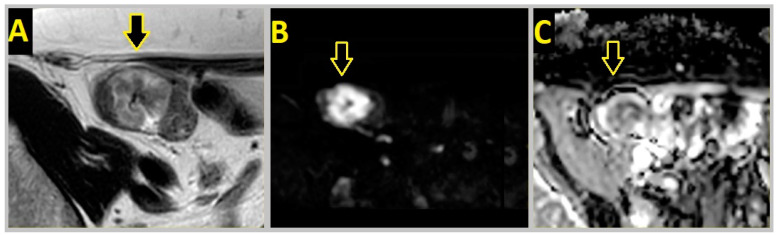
A 78-year-old woman with a tumor (marked by a yellow arrow) in the ascending colon as identified with colonoscopy. (A): Axial T2-weighted MRI image of the polyp-shaped tumor. (B): b1000 MRI image showed a strong signal. (C): Apparent diffusion coefficient map. The tumor’s mean apparent diffusion coefficient (delineation not shown) was 1110 × 10^−6^ mm^2^/s.
